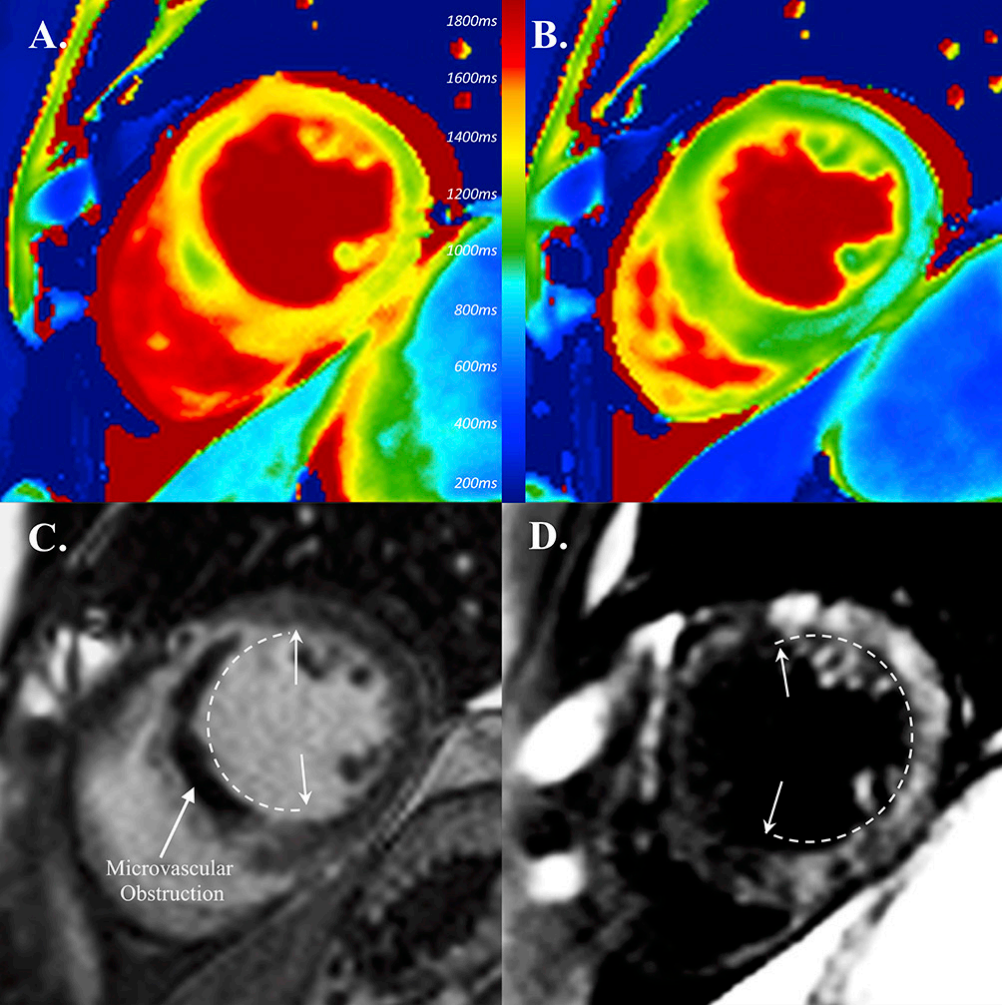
Clinical manganese-enhanced MRI (MEMRI) in a patient with myocardial infarction. Clinical tissue characterisation acutely post-myocardial infarction with native T1 mapping (A), MEMRI T1 mapping (B), gadolinium delayed-enhanced MRI (C) and manganese enhancement (D). Note shortened T1 in remote myocardium on MEMRI compared with native T1 mapping (A, B). Inversion recovery images demonstrate shortening in infarct with gadolinium (C) and in remote myocardium with manganese (D) (extent of enhancement indicated by arrows and dashed lines).
